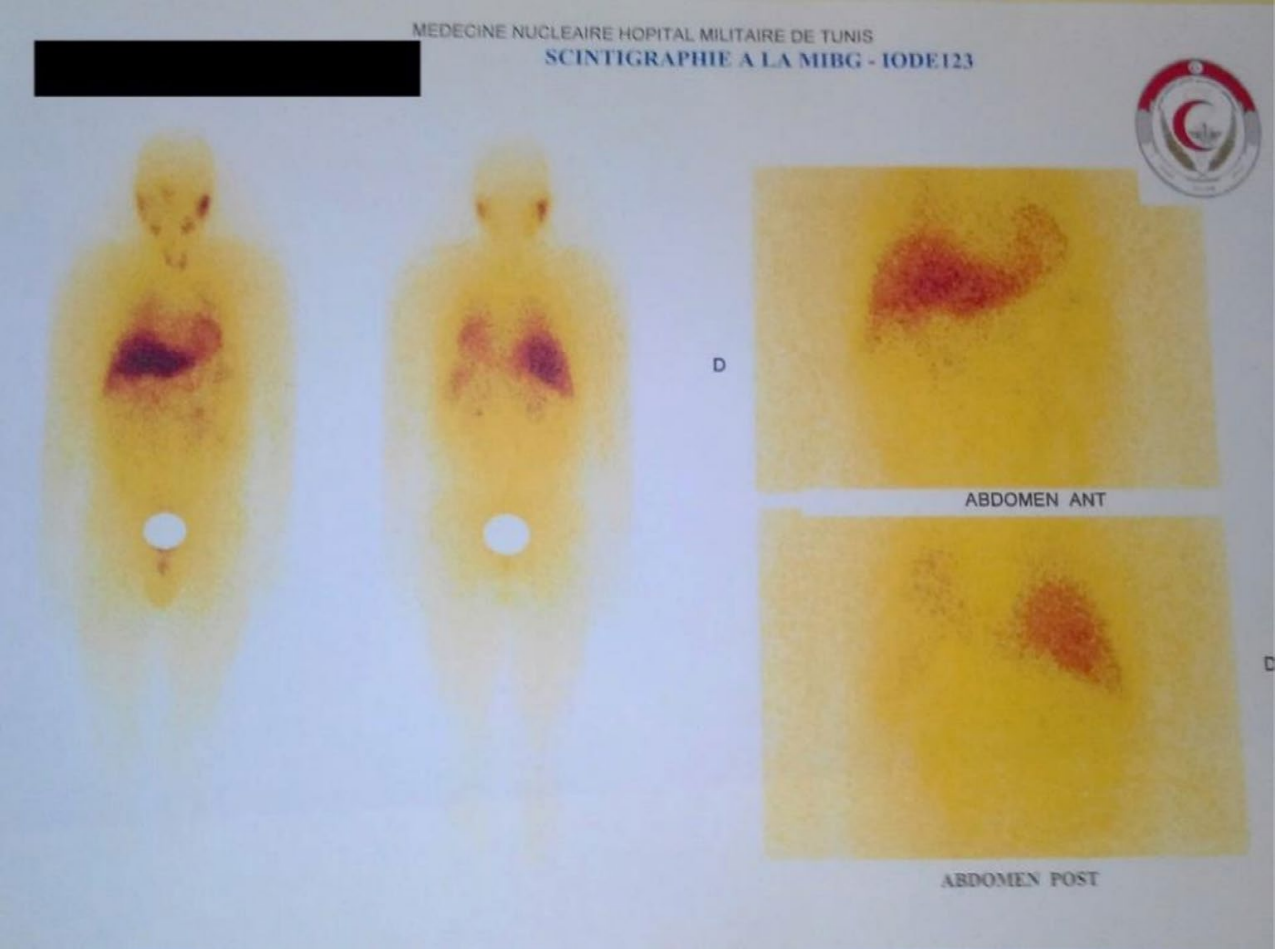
MIBG scintigraphy: No suspicious uptake, with physiological tracer visualization in the salivary glands, heart, and liver.
